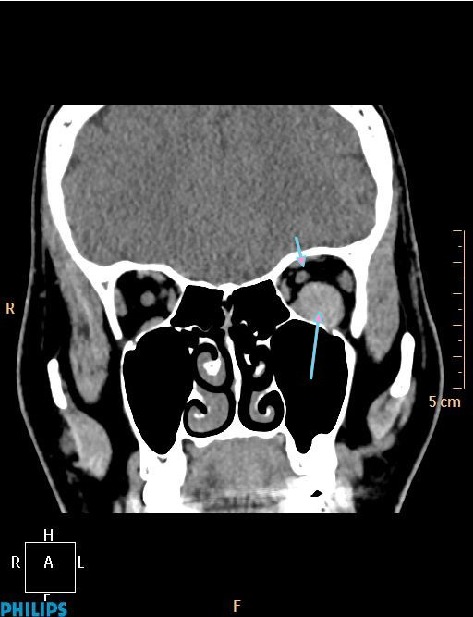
Coronal noncontrast CT image showing hyperdense haematoma (long arrow) and superomedially displaced left optic nerve (short arrow).
